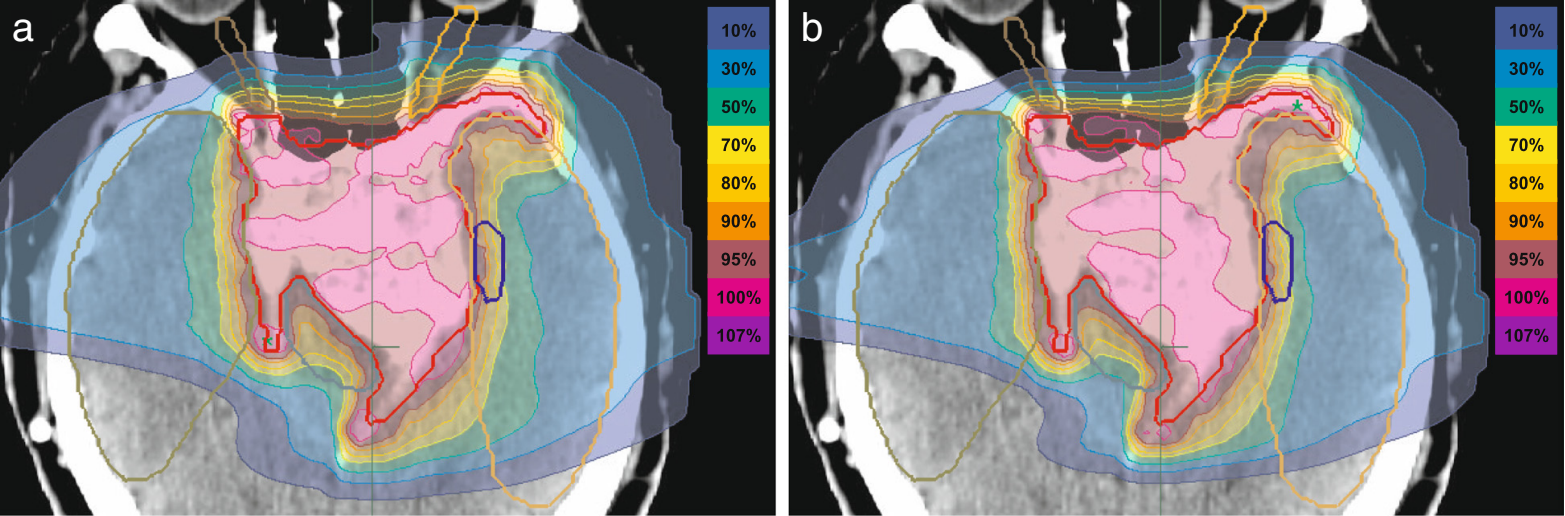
Dose distribution in a representative axial CT slice of a selected patient for the IMPT plans created using two distances between nozzle and treatment isocenter: a) 100 cm (d_ISO_) and b) 60 cm (d_ISO-40_). The reduced distance between the nozzle and patient leads to a sharper lateral dose fall-off, hence to improved target conformation.
